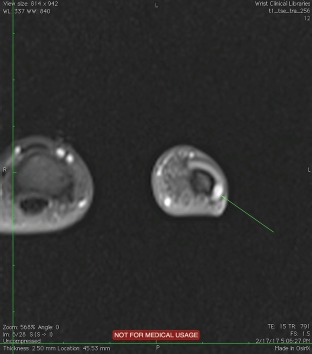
Figure 2: MRI appearance of the tumor – thumb section view
